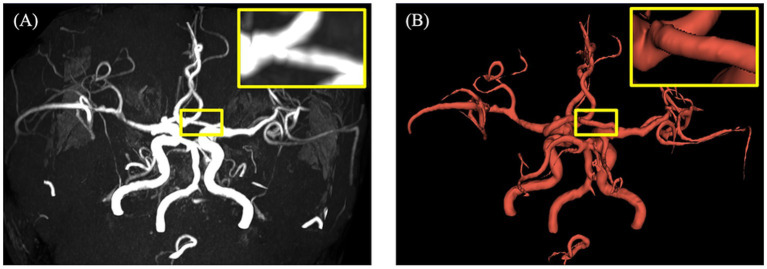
Representative case demonstrating the impact of MRA reconstruction quality on the agreement between Transcranial Doppler (TCD) and Dr. NEAR flow (DNF). (A) Original MRA maximum intensity projection image showing heterogeneous signal intensity in the right anterior cerebral artery. (B) Corresponding DNF-derived three-dimensional vascular reconstruction.
